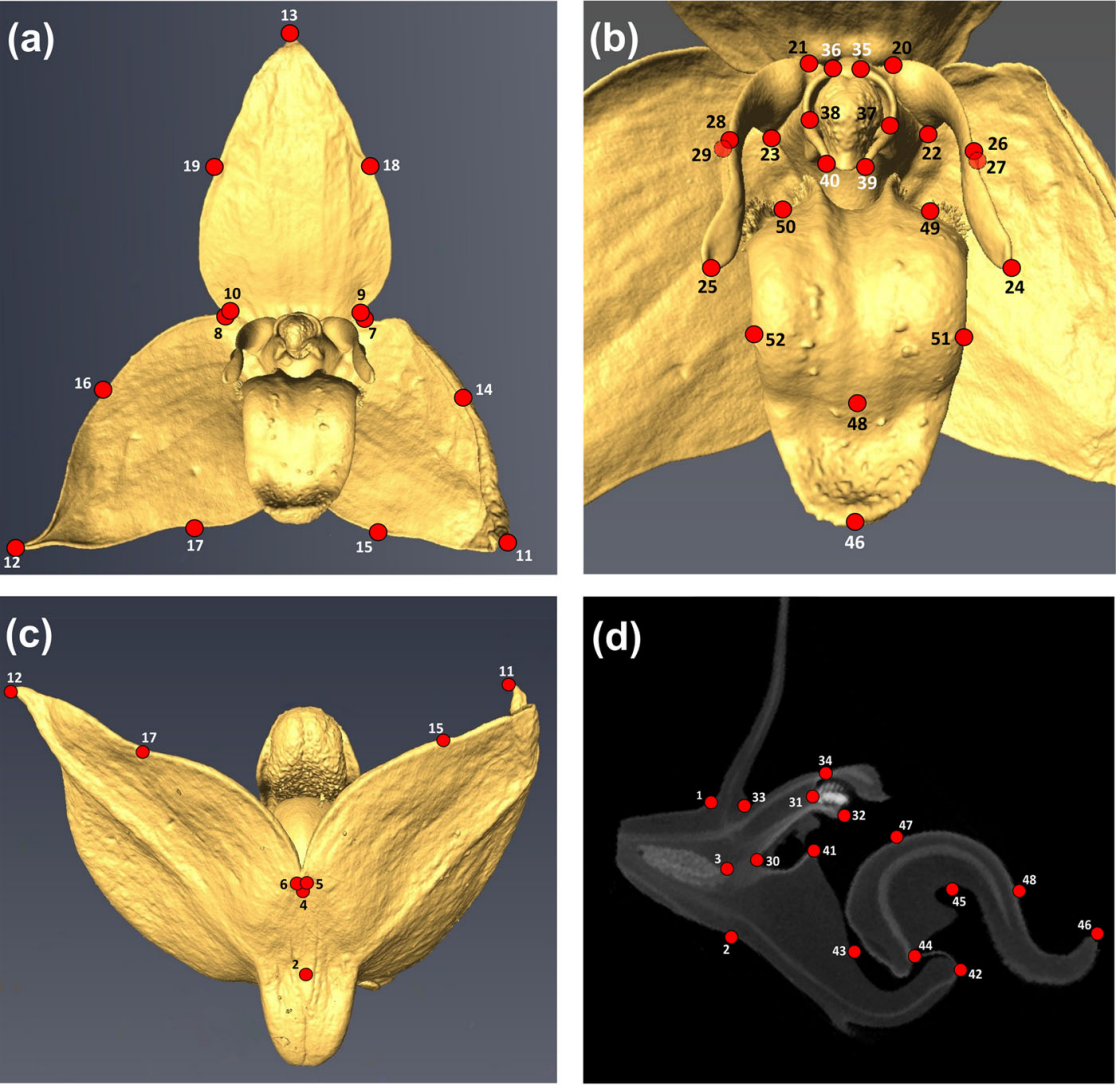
Figure 2. Landmarks (LMs 1–52; red dots) placed onto high‐resolution X‐ray computed tomography (HRX‐CT) scans of an exemplary flower of Malagasy *Bulbophyllum* (*B. francoisii* of sect. *Elasmotopus*) (see also Artuso et al. 2021). (a) Front view of resupinate flower at anthesis, in more or less horizontal orientation. (b) Close‐up of frontal view. (c) Flower seen from below (abaxial side). (d) Median (longitudinal) section. Refer to Table S2 for description of LMs and their assignment to different modules per evolutionary modularity hypothesis.
